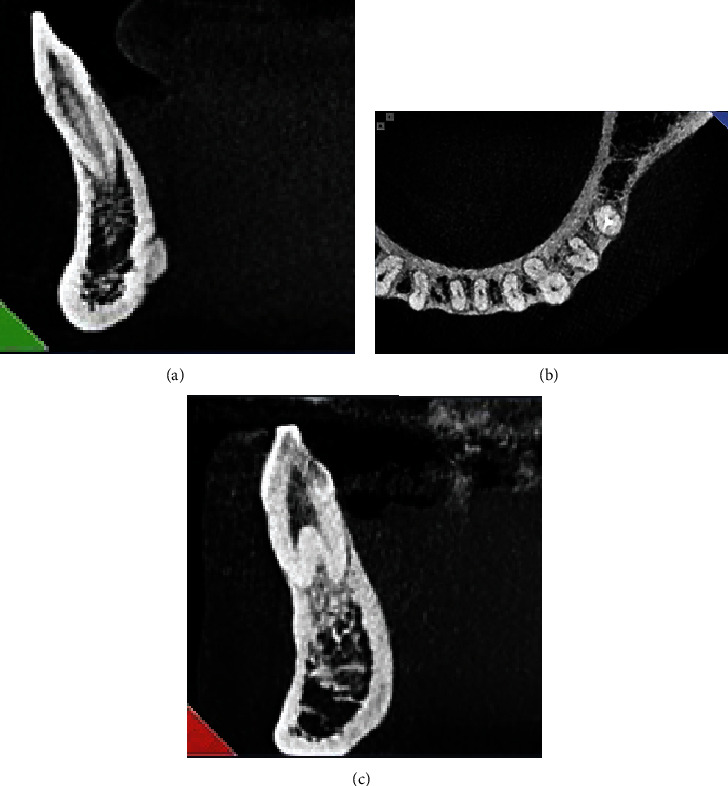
(a) Sagittal, (b) axial, and (c) coronal views of the CBCT image to evaluate the configuration of the root canal.
